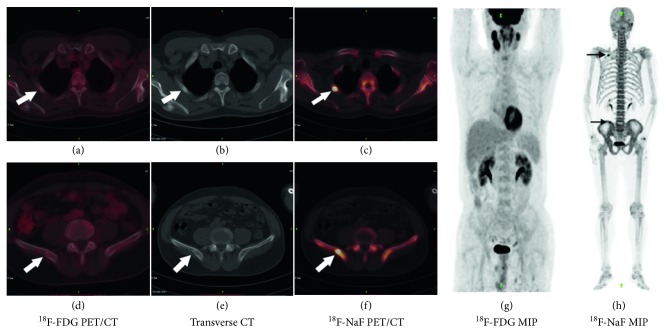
A, D, and G are parts of ^18^F-FDG PET/CT and C, F, and H are parts of ^18^F-NaF PET/CT. B and E are transverse sections of low-dose CT. Abnormal uptake of ^18^F-NaF is shown at the right rib and right ilium, whereas no abnormal concentration of ^18^F-FDG is found (arrows). The lesions are verified as osseous metastases by CT follow-up.
